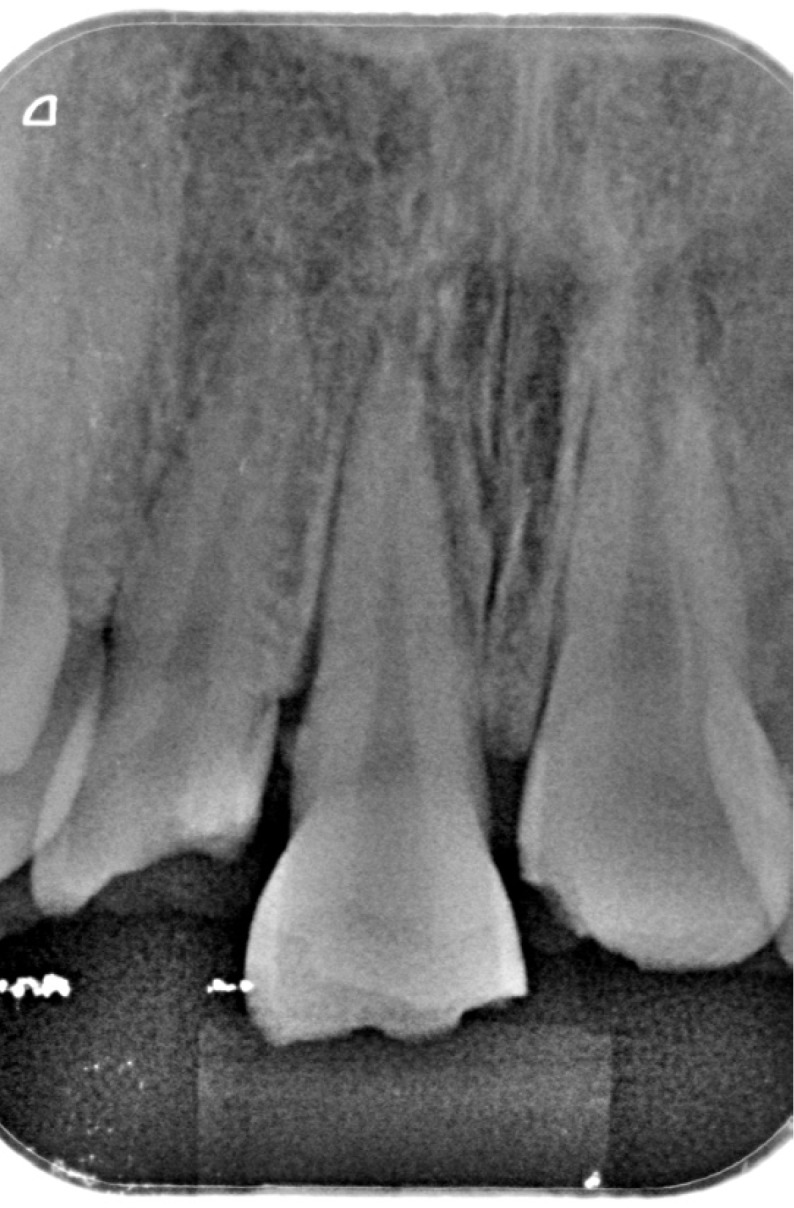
Periapical X-ray view of the upper central incisors, showing widening of the periodontal ligament of the upper right central incisor secondary to extrusive luxation.
